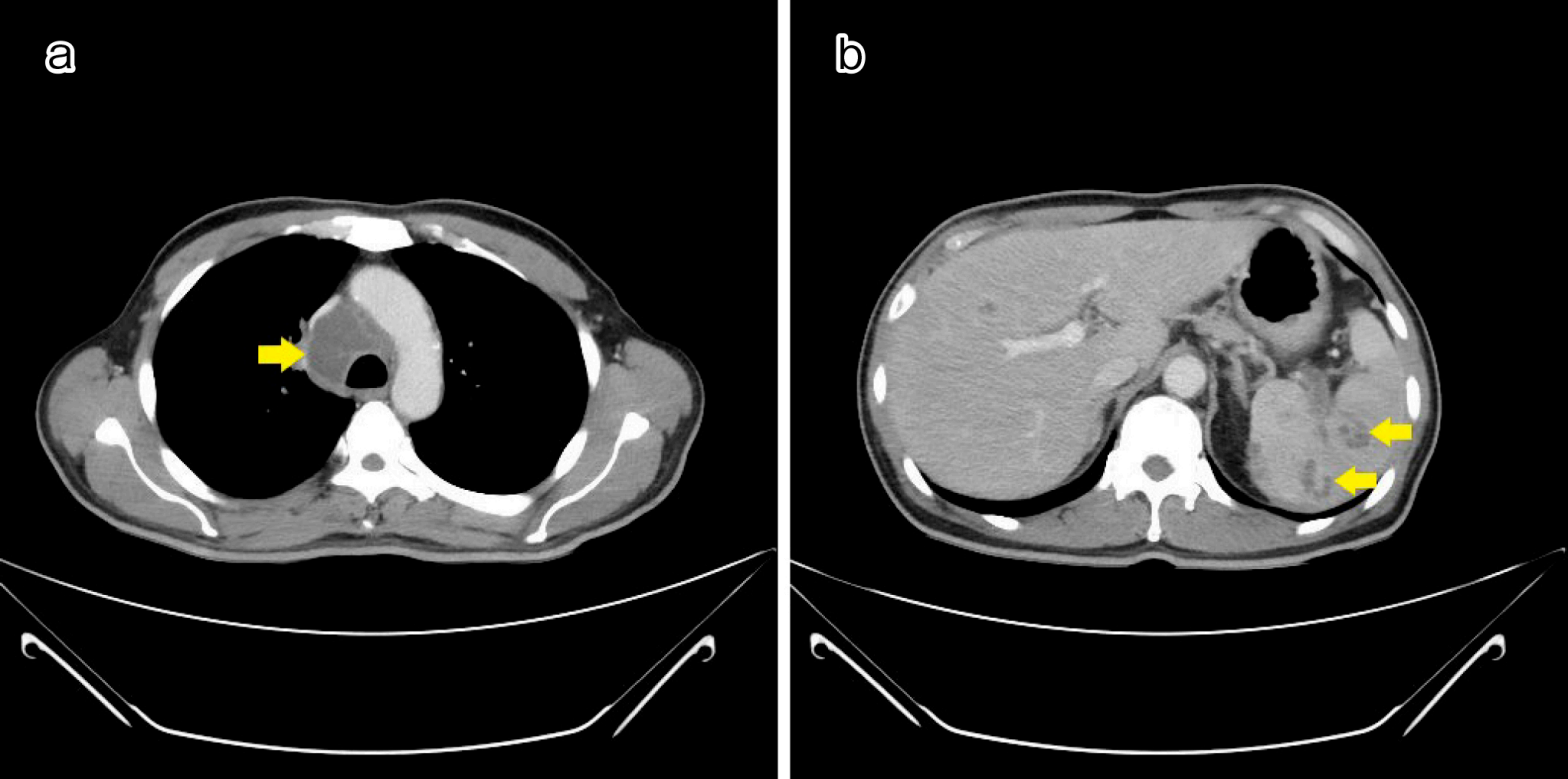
A computed tomography scan showing (a) superior mediastinal abscess and (b) multiple splenic abscesses.
